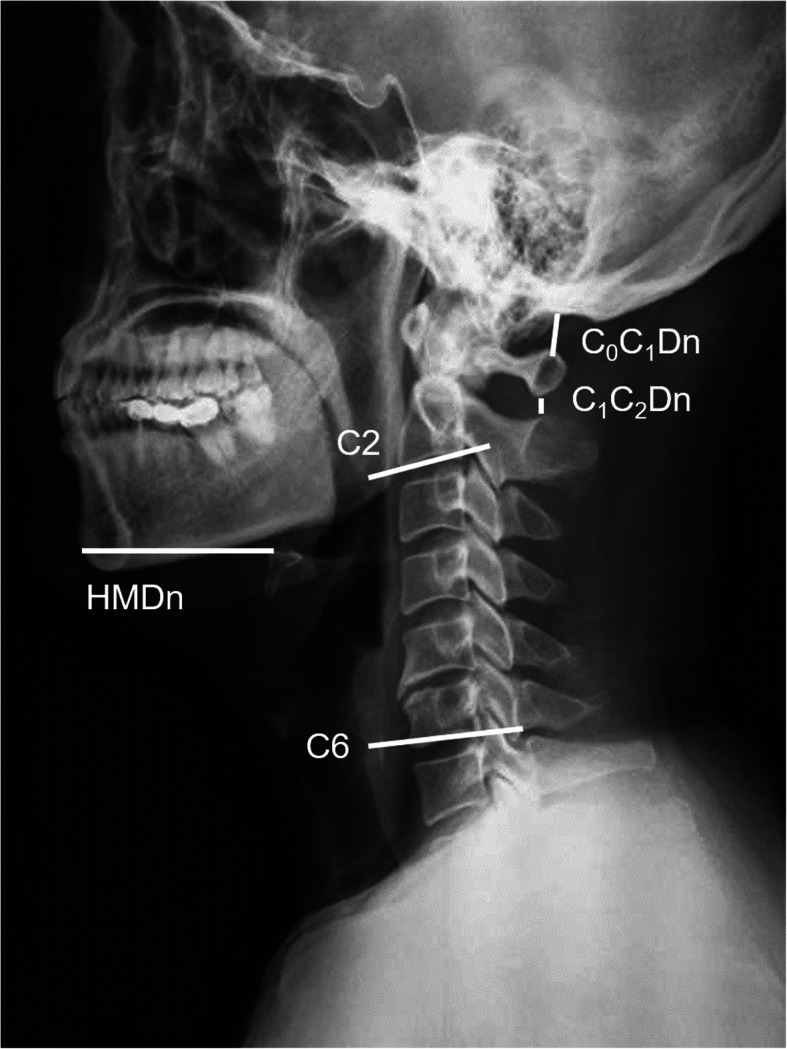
Lateral cervical X-ray film in the neutral positions. HMDn, the distance between the hyoid bone and the tip of the chin in the neutral position; C_0_C_1_Dn, the distance between the occipital bone and first cervical vertebra in the neutral position; C_1_C_2_Dn, the distance between the first cervical vertebra and the second cervical vertebra in the neutral position
